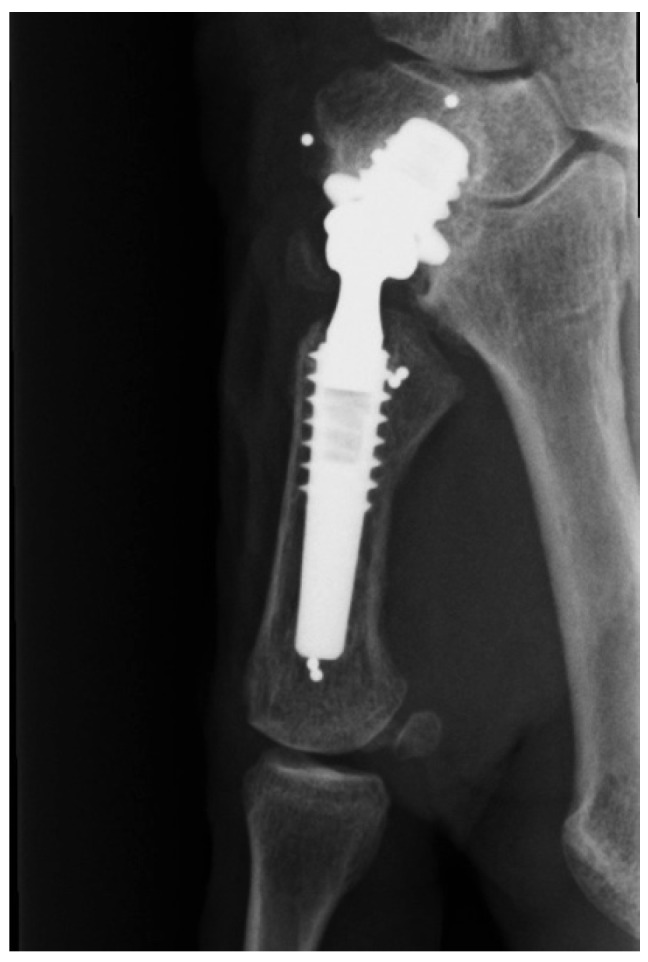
Radiograph without signs of implant failure after 29 months.
